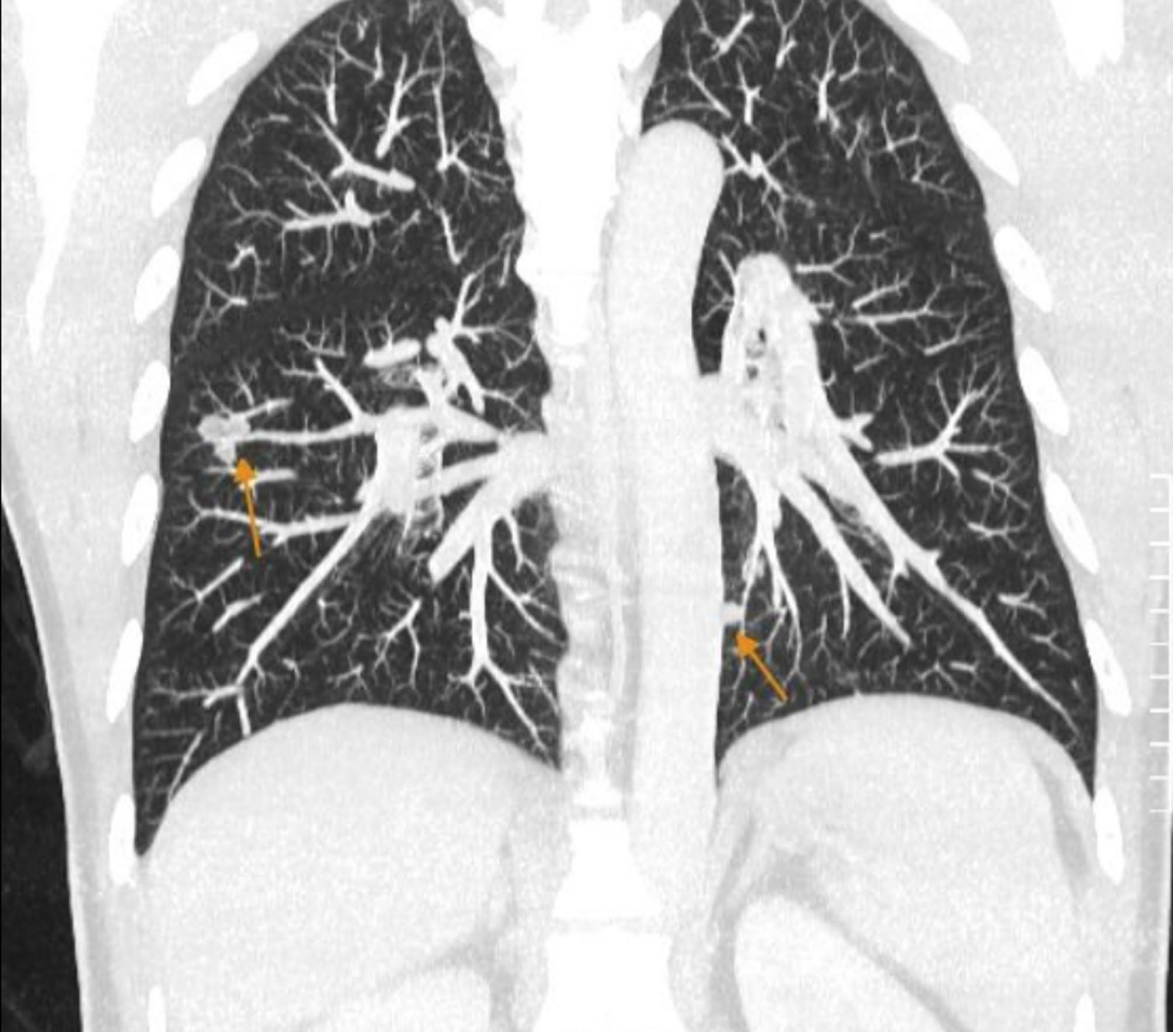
CT chest scan showing bilateral pulmonary nodules
